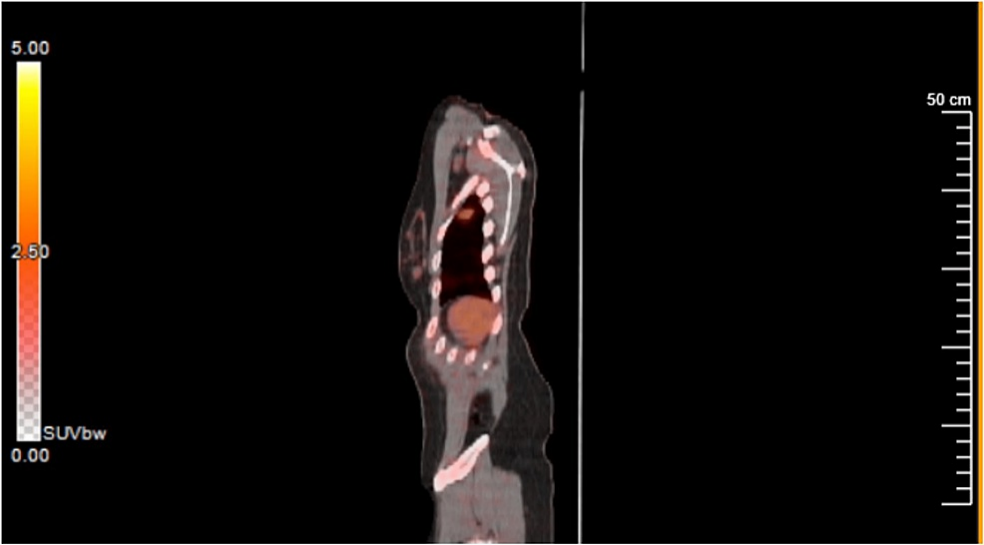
A subpleural pulmonary nodule is seen in the left upper lobe with an SUV of 2.13. Physiological uptake is also seen within the abdomen.
